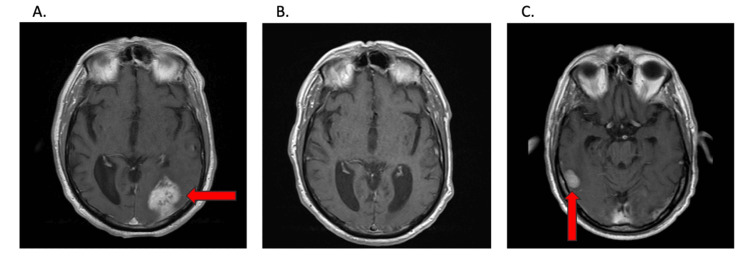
Axial T1-weighted MRI with contrast. (A) Hyperdense in the left occipito-temporal lobe marked with an arrow. (B) The reduction of the left occipito-temporal mass after initiating immunotherapy. (C) New MCC met in the right temporal lobe five months after starting treatment marked with an arrow. MCC: Merkel cell carcinoma
